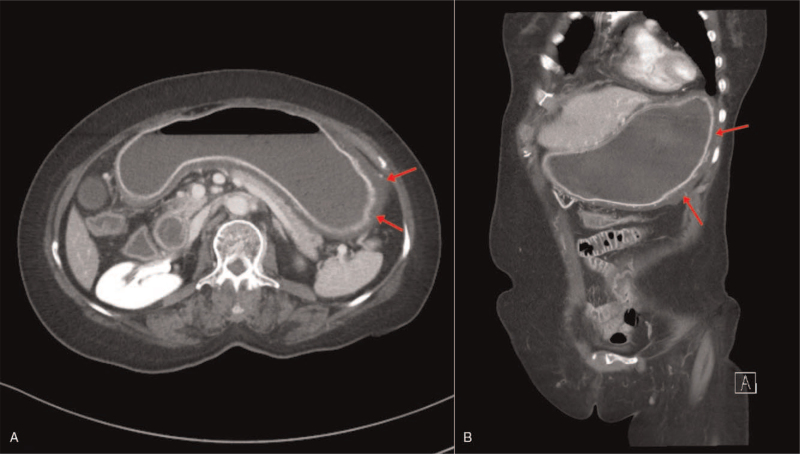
The first abdominal computed tomography performed at the emergency department showed luminal distention and wall thickening of the entire stomach (red arrows).
